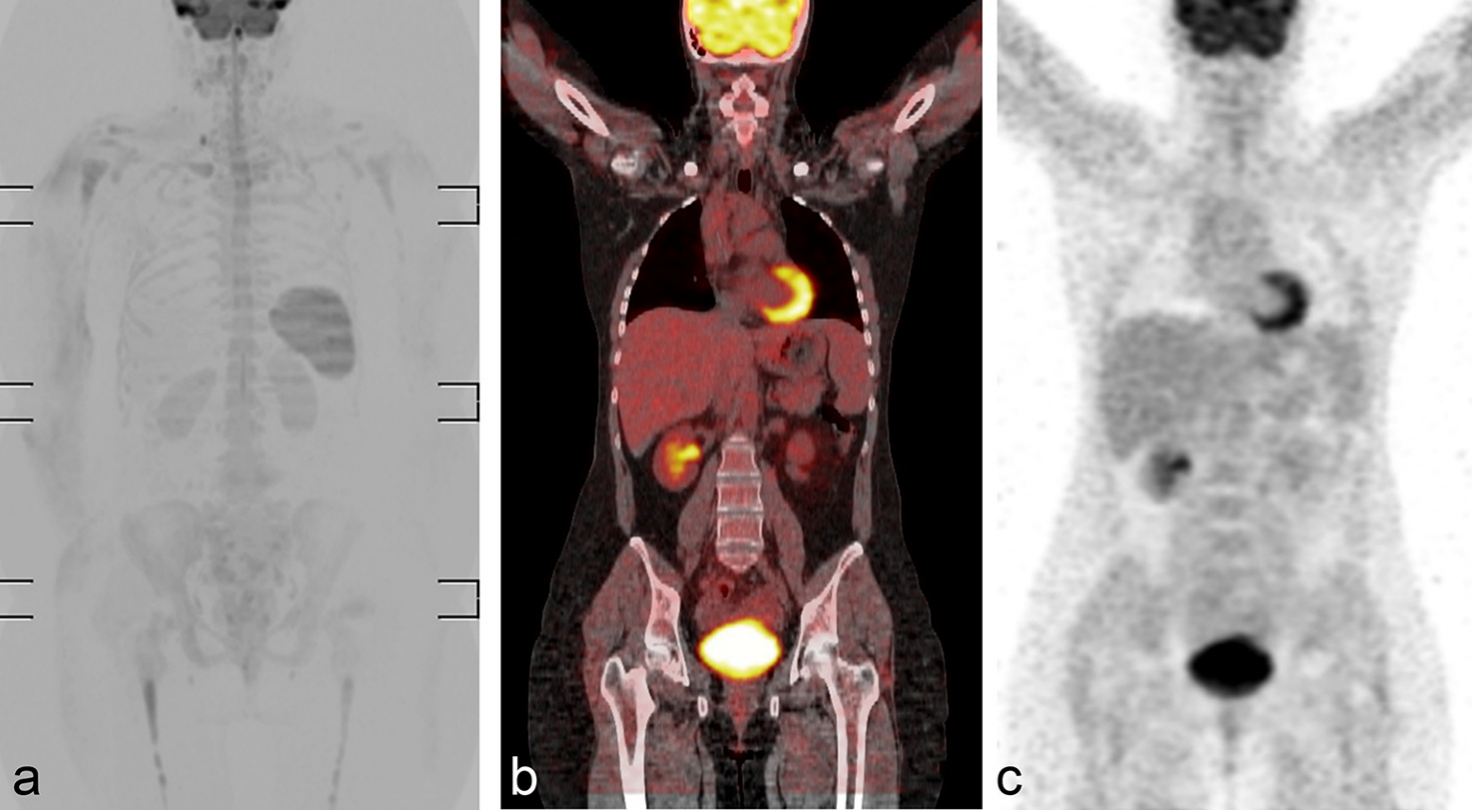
Images from scanning performed 1 month after the end of bleomycin, etoposide, doxorubicin, cyclophosphamide, vincristine, procarbazine and prednisone regimen. Coronal maximum intensity projection diffusion-weighted (a), fludeoxyglucose- positron emission tomography/CT (b) and fludeoxyglucose-positron emission tomography (c) images showing complete remission of the disease.
